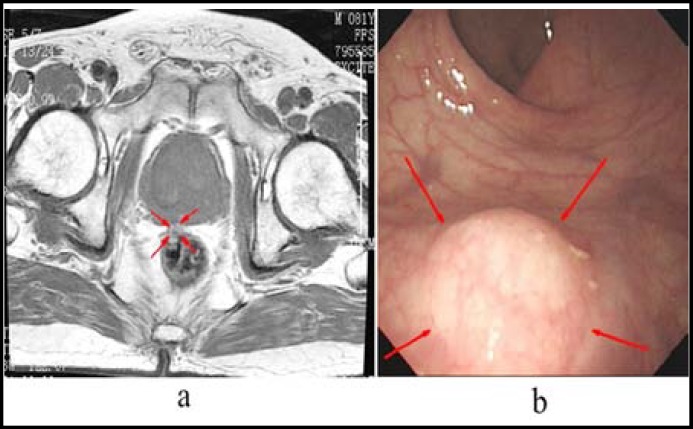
a. Colonoscopy shows the nodule (arrows), it was 1cm in diameter and in the anterior rectum 5cm above the anal verge with an otherwise normal look of the surface rectal mucosa. b. Axial T1-weighted MRI demonstrates a low intensity and well-circumscribed nodule (arrows) measuring 1cm in diameter at the level of 5cm above the anal verge protrude to the rectal lumen.
